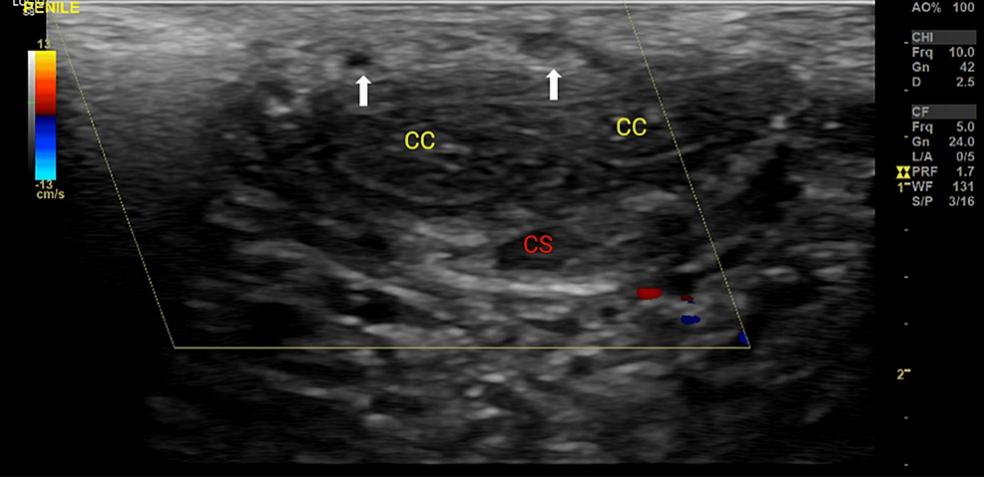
Penile color Doppler ultrasound: transverse section Yellow arrow: Corpus cavernosum (CC) of the penis; Red arrow: Corpus spongiosum (CS) of the penis. White arrow: Thrombosis of the superficial dorsal vein of the penis without flow.
